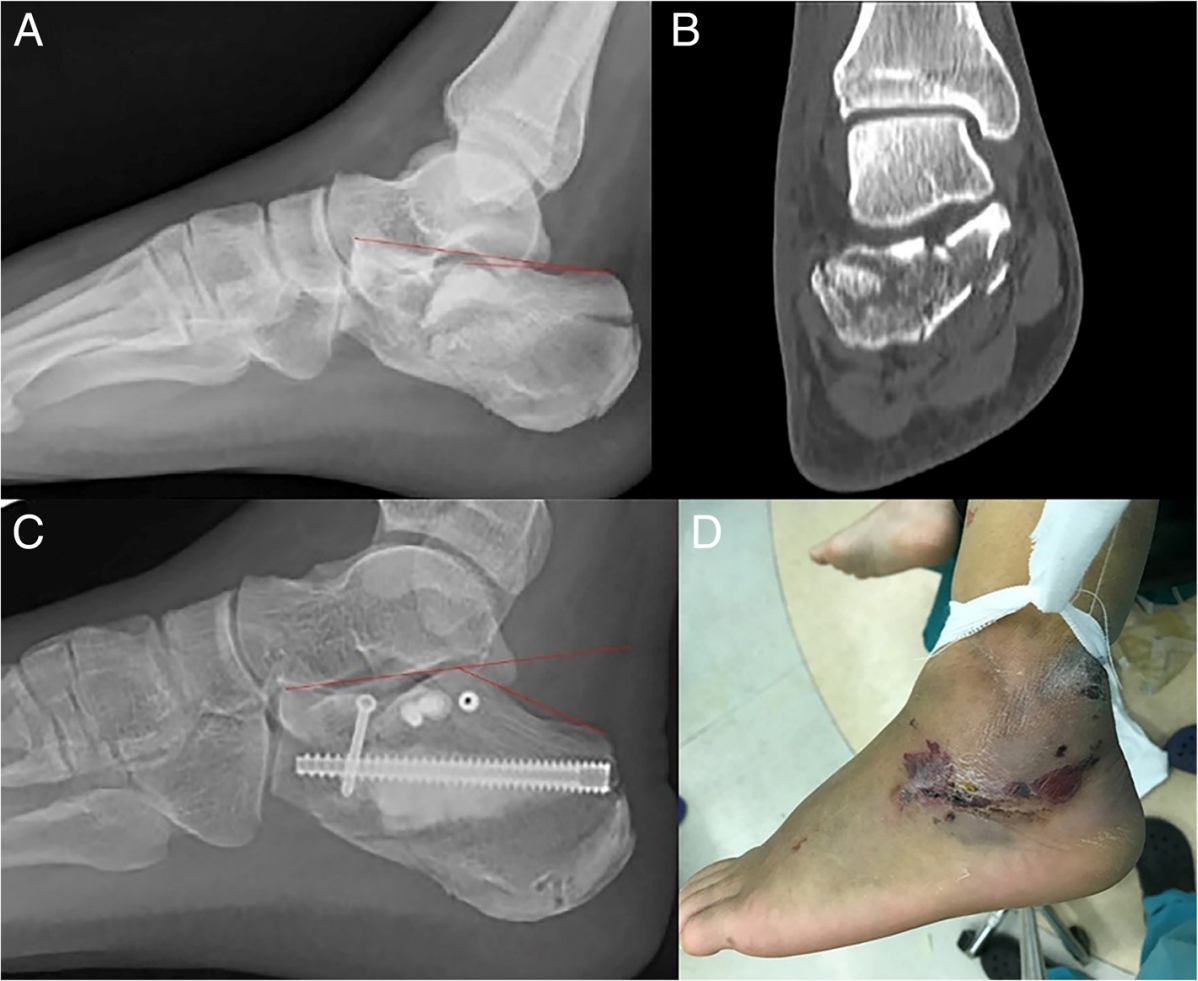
Case 1: A 40-year-old male with diabetes and severe soft tissue injury. X-ray (a, c) showing the lateral view of the calcaneus fracture before and after closed reduction percutaneous fixation surgery. The Bohler’s angle (red line) was reduced from − 3 to 28°. CT scan (b) showing a comminuted fracture in the articular surface (Sanders type IV). Bad soft tissue situation of calcaneous skin (d)
